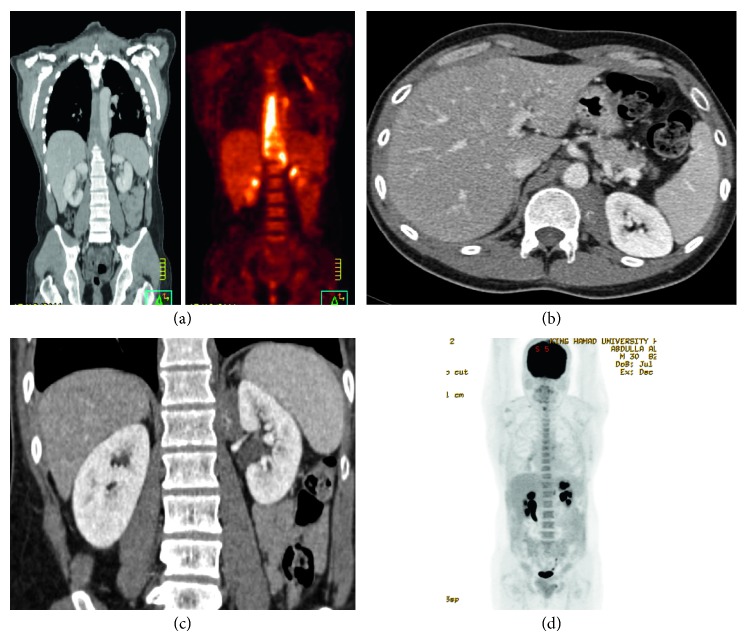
(a) PET CT of 2011 revealed a soft tissue paravertebral mass extending from the T7 till L1. (b, c) Contrast-enhanced computed tomography (CT) scan showing para-aortic soft tissue thickening as well as retrocrural thickening. (d) Bilateral mild hydronephrosis.
